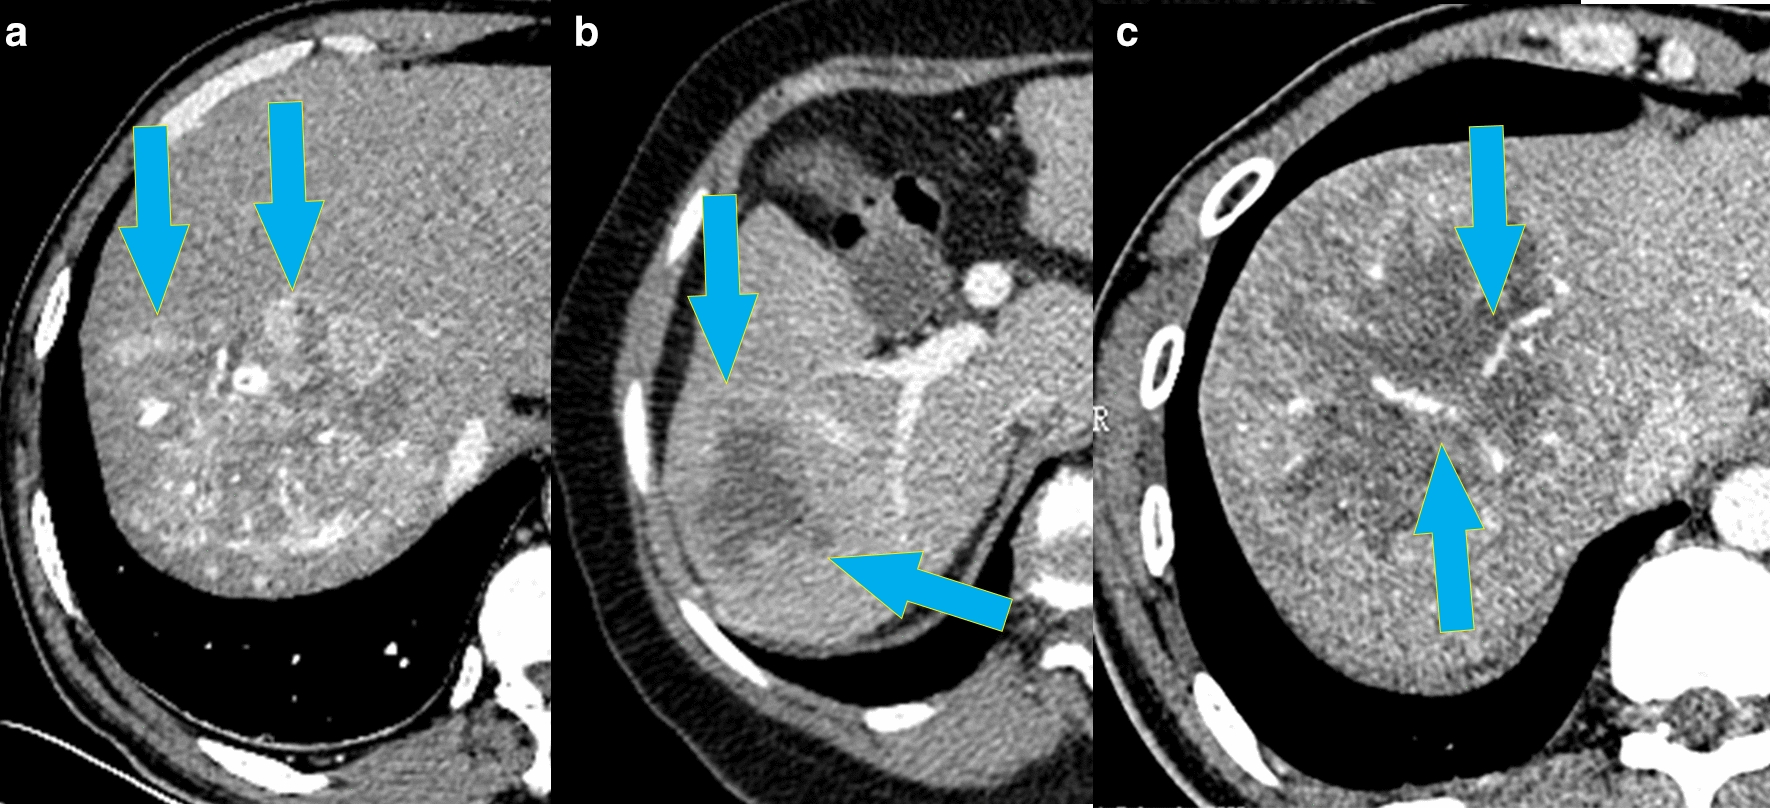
Computed tomography scan revealed the patients with different RFS. a peritumoral enhancement: recurrence 3.2 months, external portion enhancing in the arterial phase (arrows). b nonsmooth tumor margin positive: recurrence 4.5 months, focal extranodular extension (arrows) in the venous phase. c The two-trait algorithm predictive of MVI (TTPVI) positive: recurrence 2.8 months, blue arrows show internal artery and lower image means noncontinuous hypoattenuating halos
